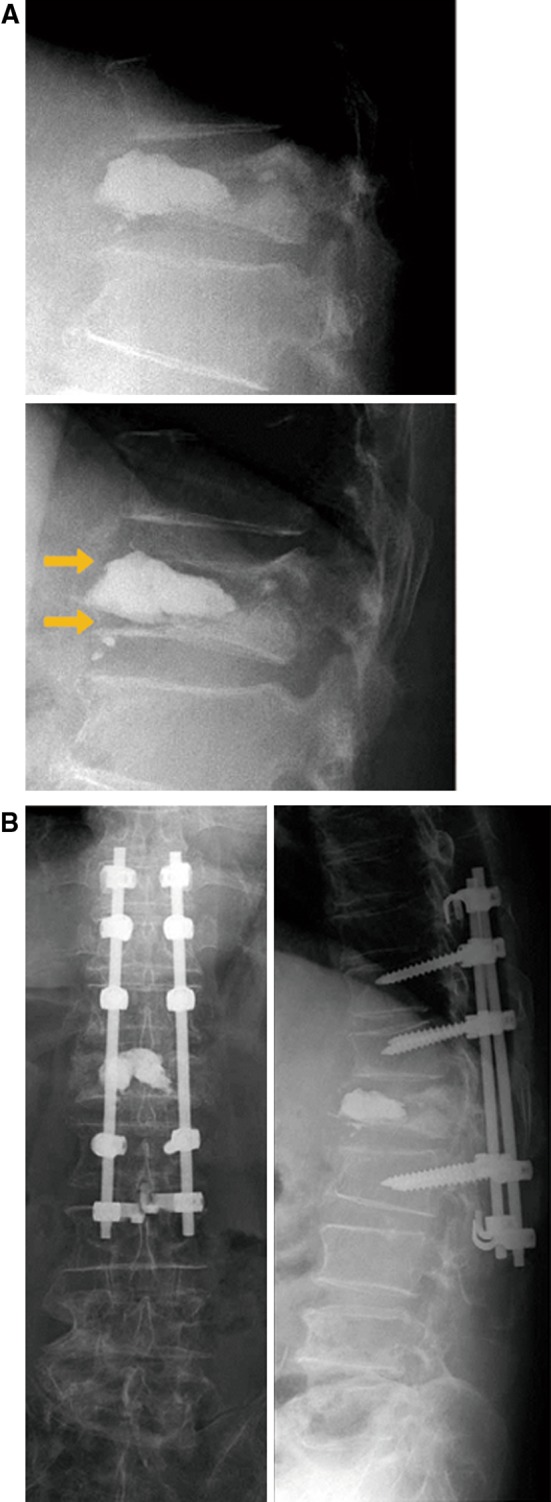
(Case 1) a Lateral spine flexion–extension radiographs demonstrated instability, and PMMA did not adhere to the endplate of the treated vertebrae. b Surgical intervention was performed by pedicle screw and hook fixation from Th10 to L2
